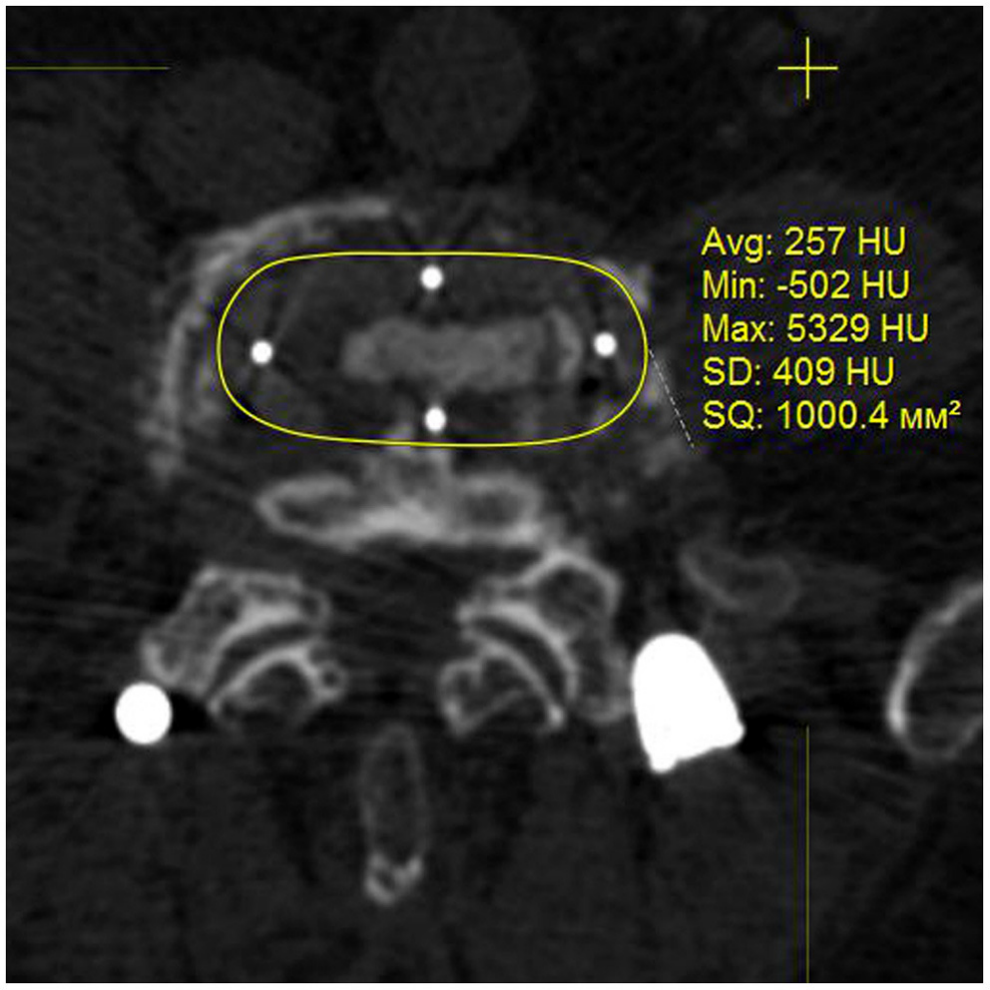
Cage used for DLIF, postoperative CT image in axial plane.
